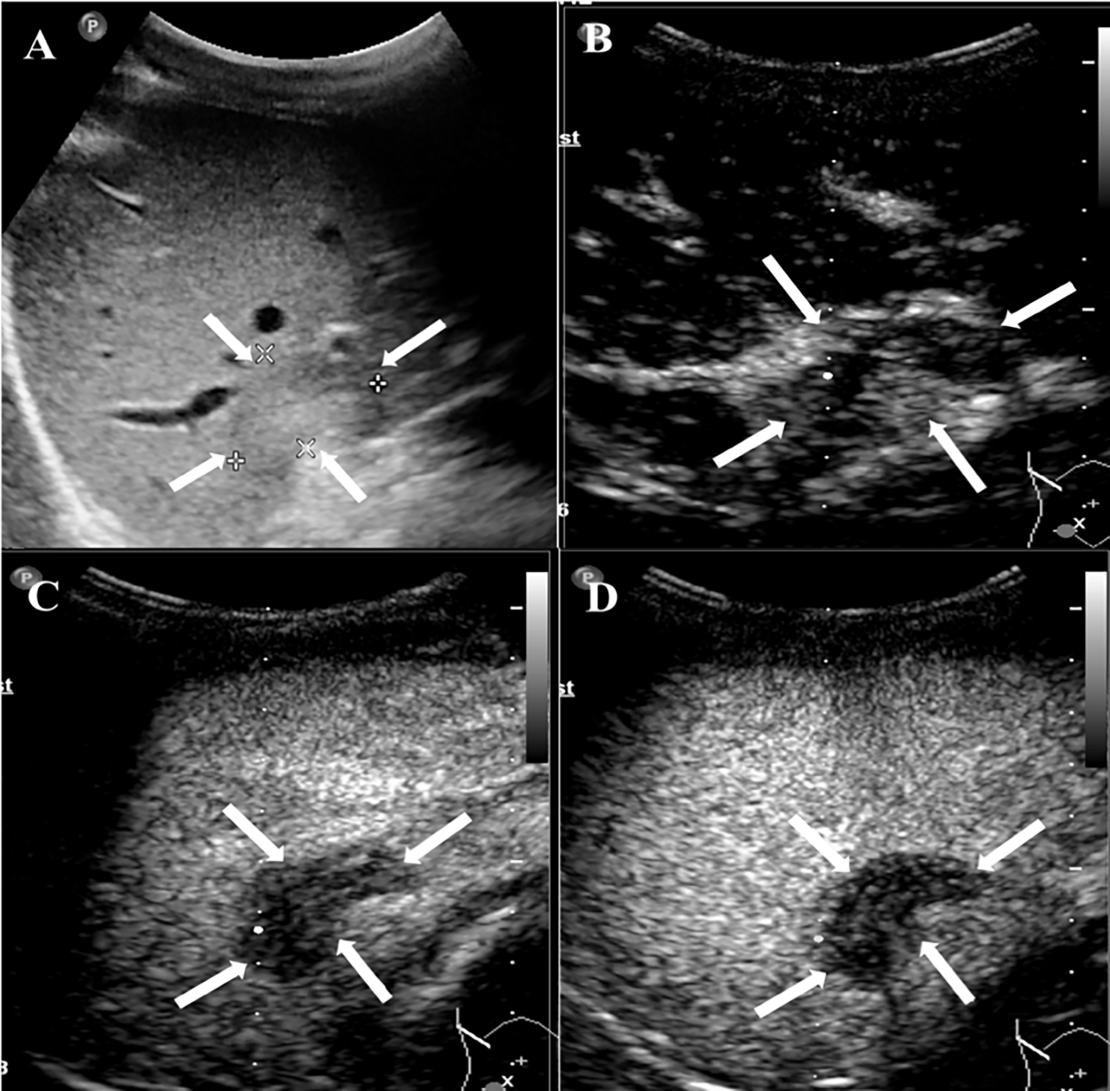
CEUS features of HEHE in a 24-year-old male. (A) Greyscale ultrasound presented a subcapsular hypoechoic lesion (arrows) in the right hepatic lobe. (B) This lesion showed peripheral rim-like slight hyperenhancement (arrows) in the arterial phase (17 s). (C) The degree of enhancement washed out fast than the surrounding liver parenchymal and displayed hypoenhancement (arrows) in the portal phase (50 s). (D) In the late phase (135 s), the lesion remained hypoenhancement (arrows).
